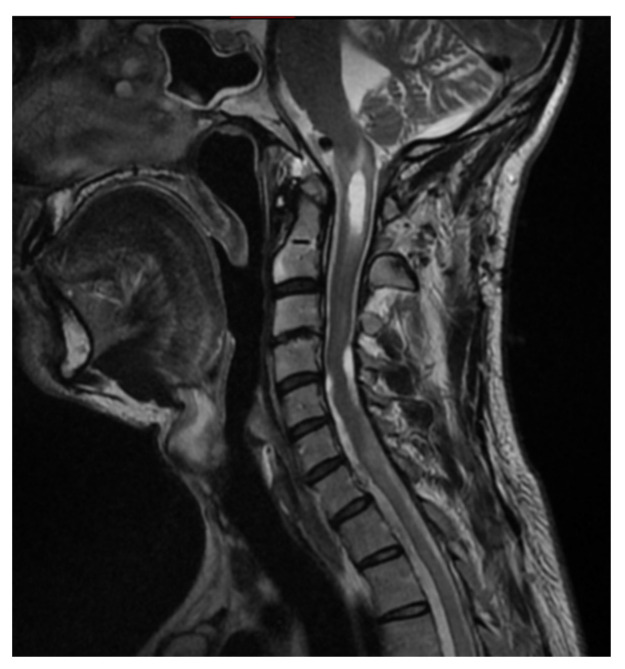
Patient 4 weeks after tumor resection with enlarged central canal of the cervical spinal cord. Single Sagittal Scan MRI T2.
